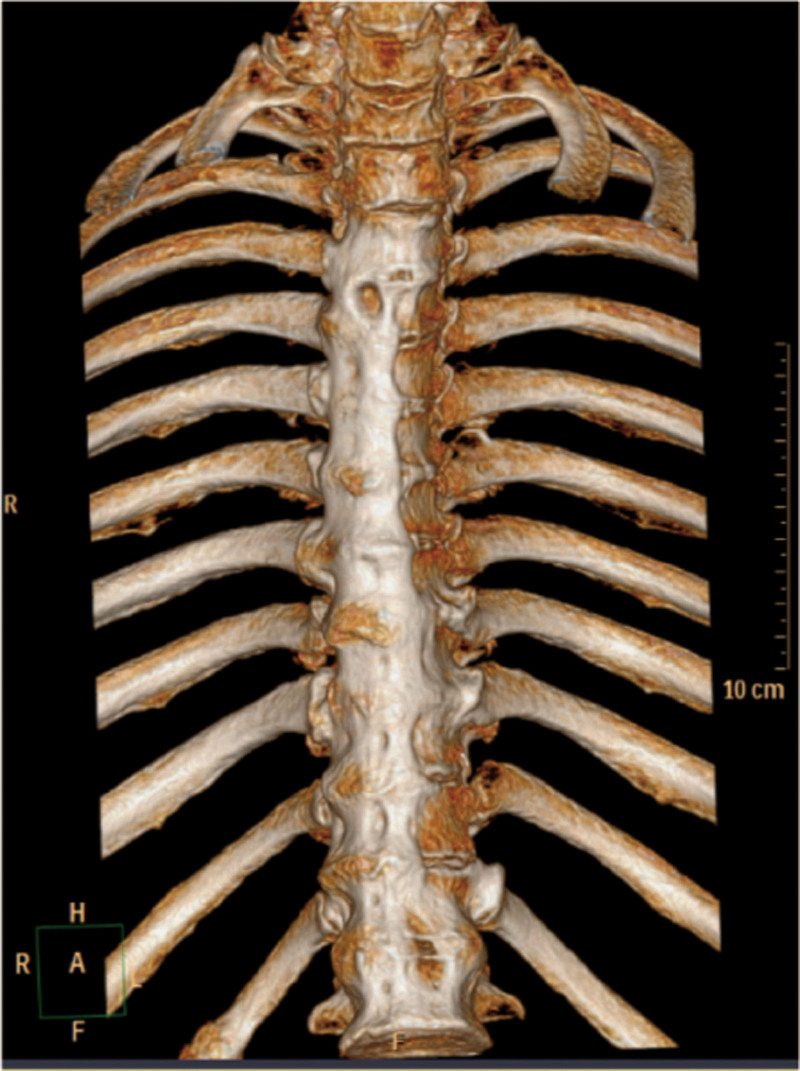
CT 3D imaging of thoracic vertebra: it can be seen that there are bright connections formed by calcification of the anterior longitudinal ligaments of multiple segments.
